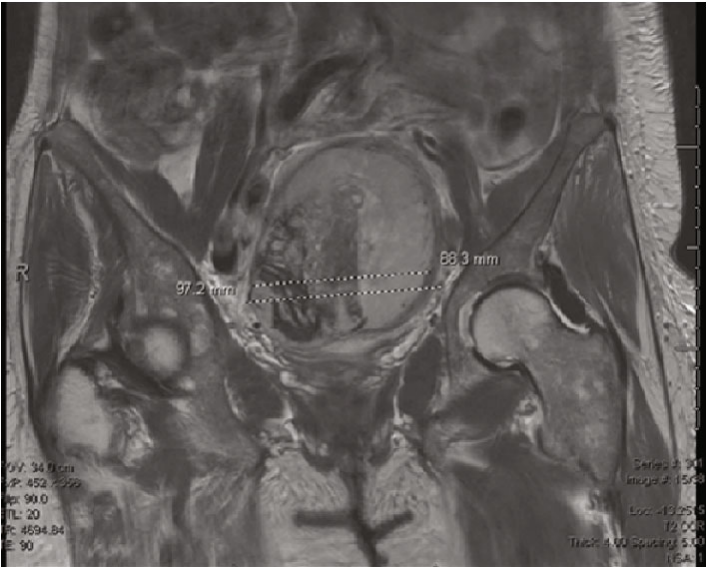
MRI pelvis depicting pelvic mass, later confirmed with pathology to be HGESS.
